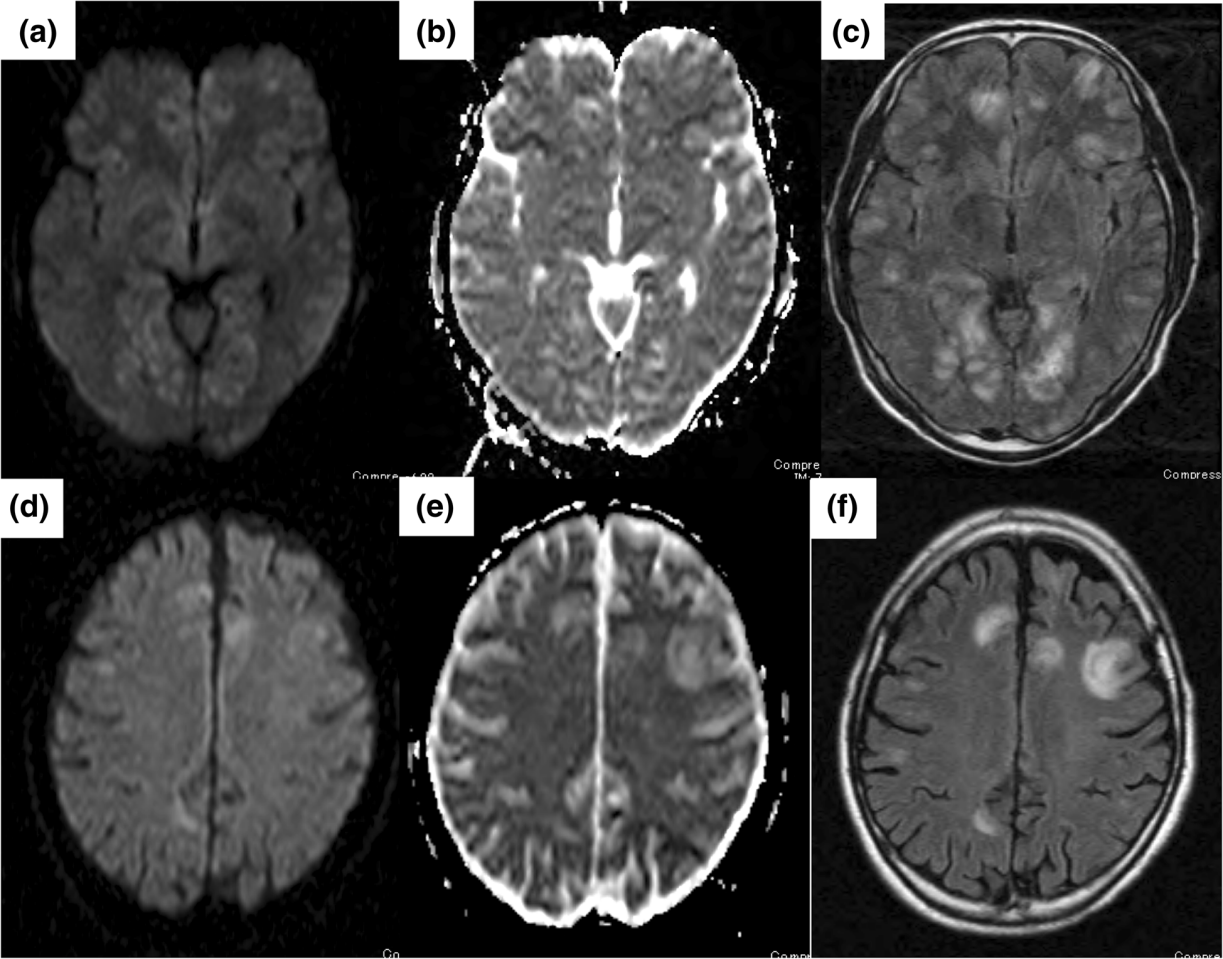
Brain magnetic resonance imaging on the day of admission and on day 261 (showing recurrence). a–c Images on the day of admission; d–f images obtained on day 261 when the patient’s central nervous system symptoms deteriorated. a and d Diffusion-weighted sequences images. b and e Apparent diffusion coefficient images. c and f Fluid-attenuated inversion recovery images. a, b, d, e show that central nervous system lesions did not derive from vasculitis and cerebrovascular diseases because those were not along the vessels. Hyperintensity in the cortex and subcortical white matter can be seen in c and f. c Margins of the lesion are clearly visualized. f Margins of the lesion are unclear
